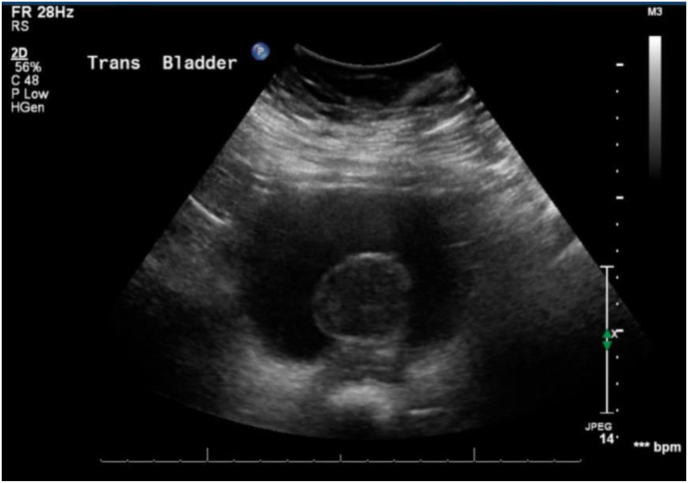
Bladder ultrasonography revealing a solid posterior bladder mass measuring 4.1 × 3.8 × 3.0 cm.
